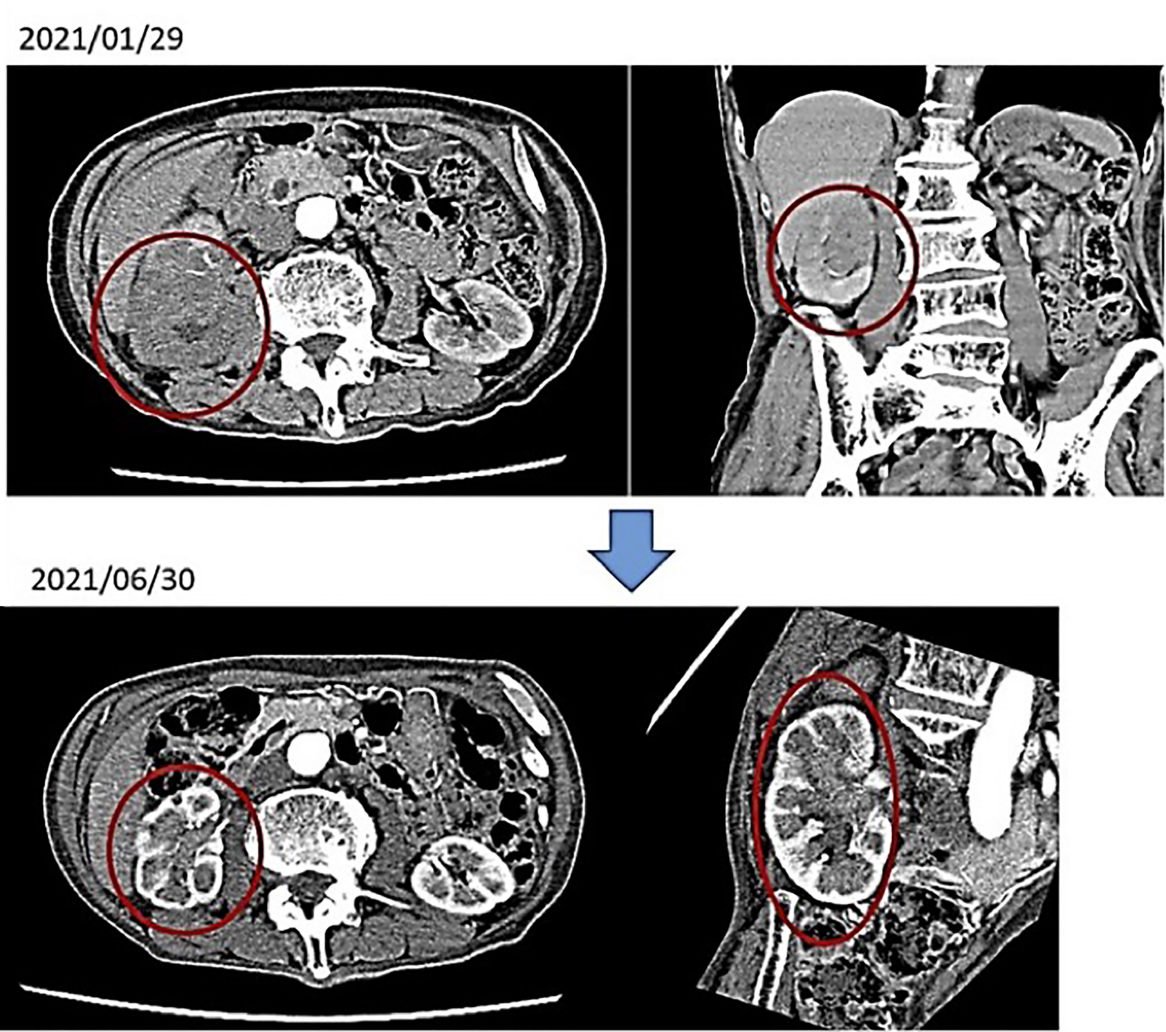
A contrast enhanced computed tomography of abdomen are shown.
